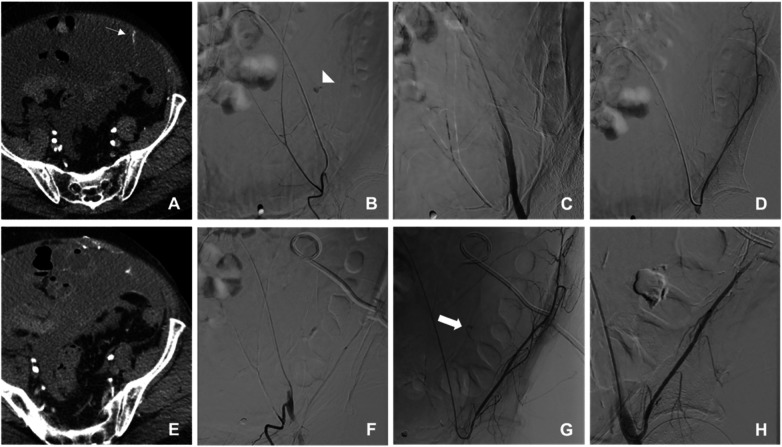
A 57-year-old patient with alcoholic cirrhosis complicated by acute-on-chronic liver failure, with clinical suspicion of iatrogenic hemorrhage following ascites paracentesis. (A) Axial contrast-enhanced CT image demonstrates active contrast extravasation arising from the left inferior epigastric artery (thin arrow). (B) Selective angiography confirms active bleeding from a branch of the left inferior epigastric artery (arrowhead), (C) which was successfully treated by embolization using n-butyl cyanoacrylate mixed with ethiodized oil in a 1:3 ratio. (D) Selective angiography of the ipsilateral deep circumflex iliac artery shows no evidence of active bleeding. (E) One day after embolization, the patient again developed clinical signs of hemorrhage and repeat contrast-enhanced CT demonstrated new active contrast extravasation in the same anatomical region. (F) Repeat angiography confirmed persistent occlusion of the left inferior epigastric artery following prior embolization, (G) but revealed new active contrast extravasation from a branch of the ipsilateral deep circumflex iliac artery (bold arrow). (H) This newly identified bleeding source was successfully embolized using n-butyl cyanoacrylate mixed with ethiodized oil in a 1:3 ratio.
